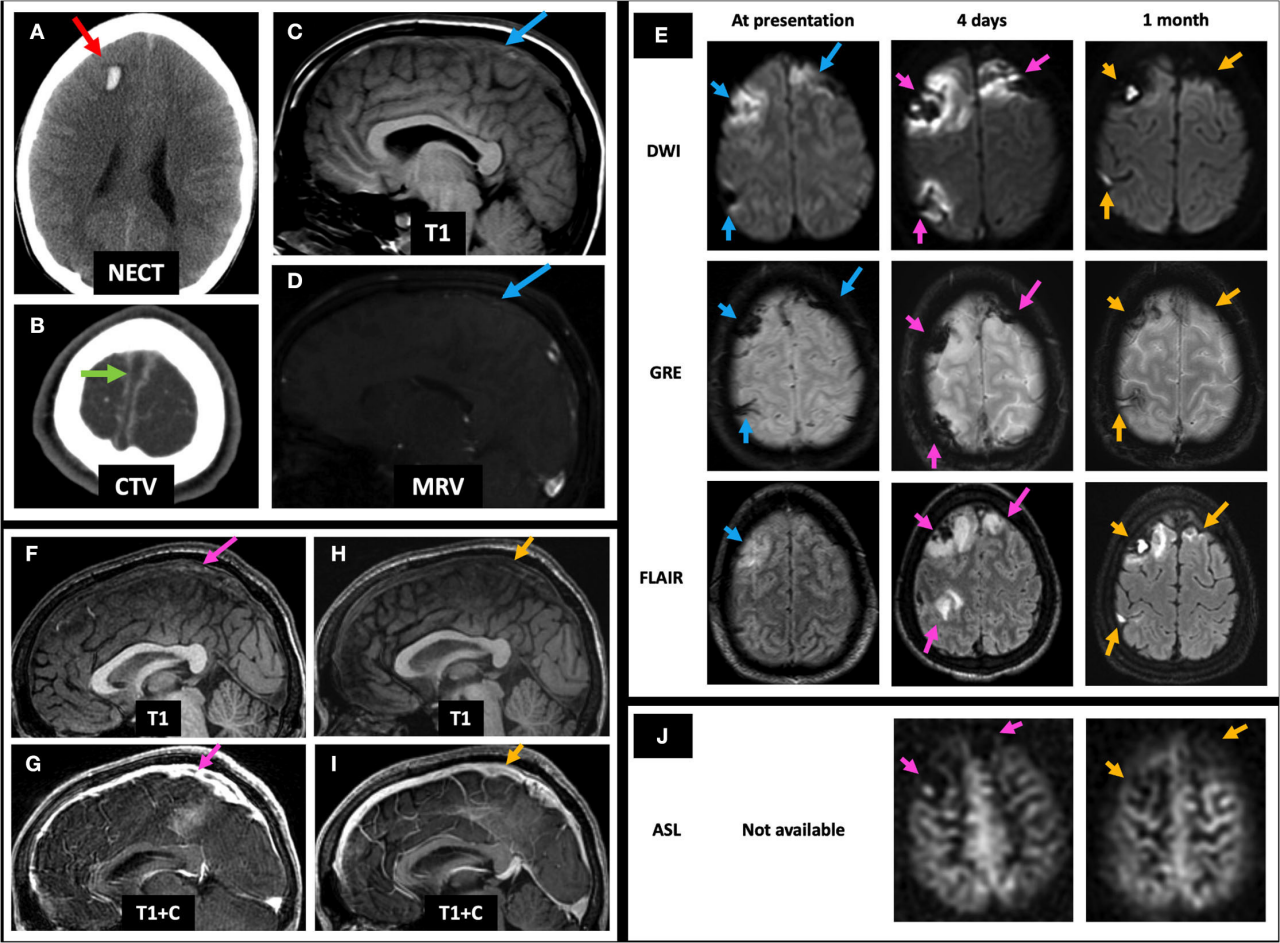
Case 2: A 23-year-old male with history of ulcerative colitis presented with headache, confusion, and agitation. Initial NECT showed bilateral frontal parasagittal hemorrhages (right parasagittal hemorrhage shown on A, red arrow). CTV showed a filling defect in the SSS (B, green arrow). Initial C-MRI/MRV showed subtle T1 isointense SSS thrombus (C, blue arrow) and no SSS flow-related enhancement (D, blue arrow). Mild bifrontal edema and hemorrhage are seen (E, left column, blue arrows). Four days later, the SSS thrombus appears mildly hyperintense (F, pink arrow) and demonstrates an empty delta sign (G, pink arrow), accompanied by slightly increased DWI and FLAIR signal abnormalities, as well as persistent GRE hypointensity (E, middle column, pink arrows). There is also associated right greater than left frontal hypoperfusion on ASL images (J, middle column). One month post-initial presentation, the SSS is again T1 isointense with resolution of filling defect (H,I, yellow arrows) and improvement in DWI, FLAIR, and GRE signal abnormalities (E, right column, yellow arrows), as well as improved but mild residual right greater than left frontal hypoperfusion on qualitative perfusion-weighted ASL images (J, right column). ASL, arterial spin labeling; CTV, CT venography; NECT, non-contrast-enhanced CT; SSS, superior sagittal sinus; C-MRI, contrast-enhanced MRI; MRV, MR venography; TS, transverse sinus.
